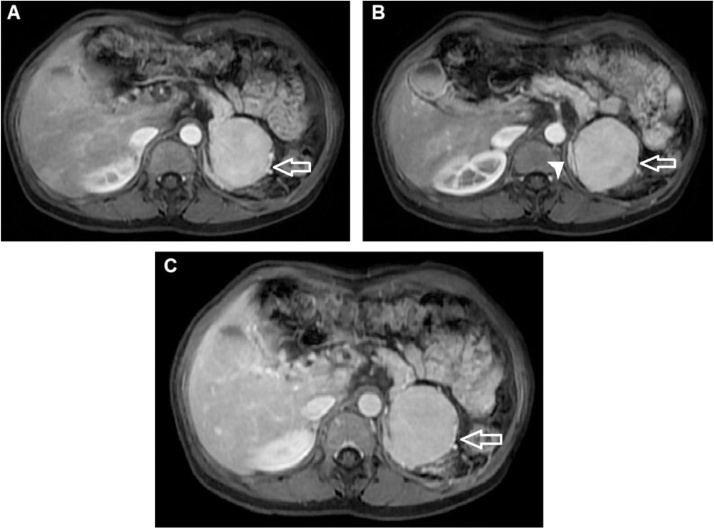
Axial T1-weighted images in the arterial phase revealed that the mass was markedly and inhomogeneously enhanced (A, arrow) and the left adrenal gland was normal (B, arrowhead). Axial T1-weighted images in the venous phase showed that the mass exhibited homogeneous enhancement (C, arrow).
